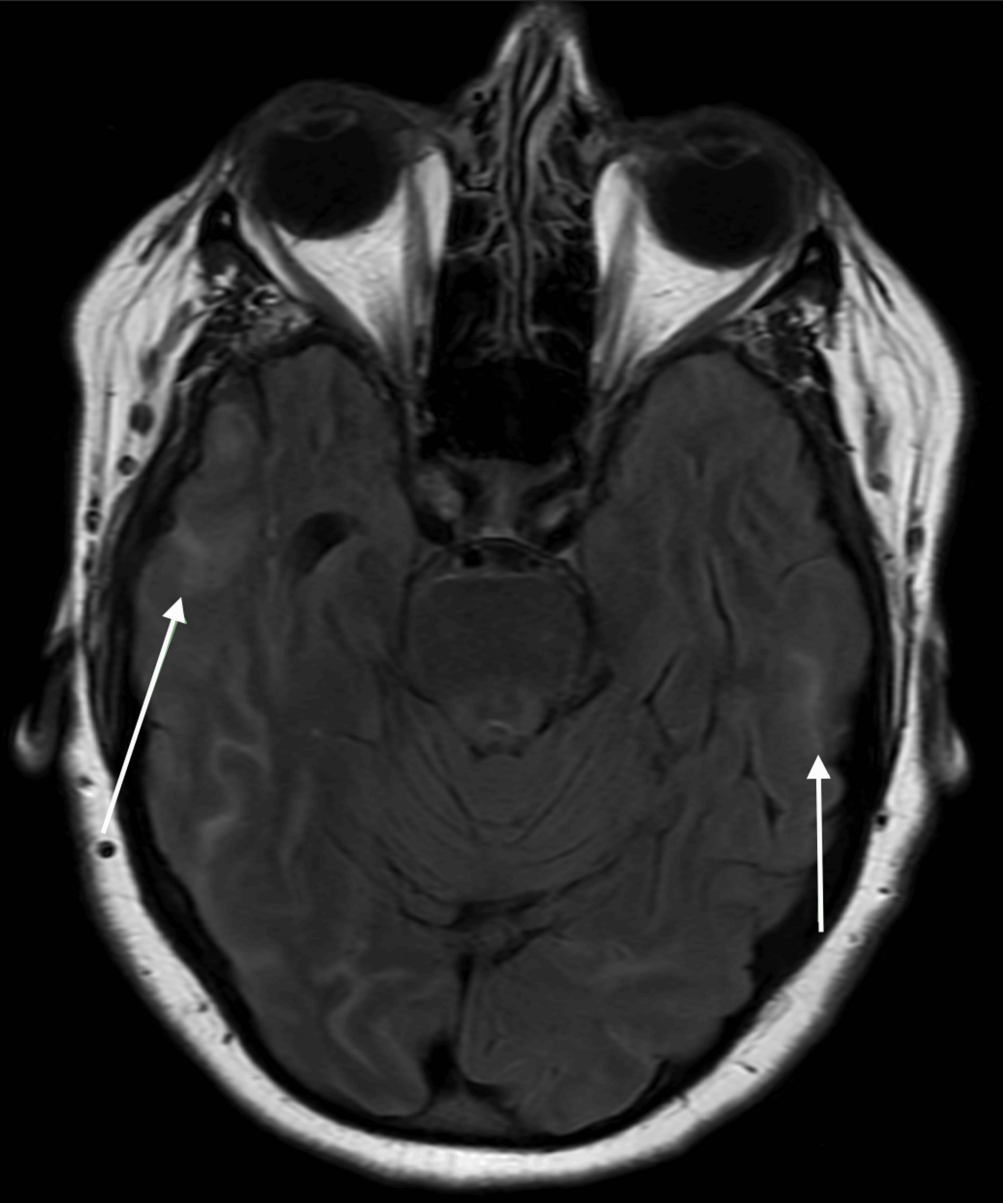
Axial T2 FLAIR MRI at the level of the temporal lobes shows bilateral curvilinear sulcal FLAIR hyperintensities (white arrows), more pronounced on the right. These gyriform signal changes are suggestive of sulcal edema, also consistent with ARIA-E in the setting of anti-amyloid therapy. No associated hemorrhage was observed on susceptibility-weighted imaging. ARIA-E: amyloid-related imaging abnormalities with edema; FLAIR: fluid-attenuated inversion recovery
